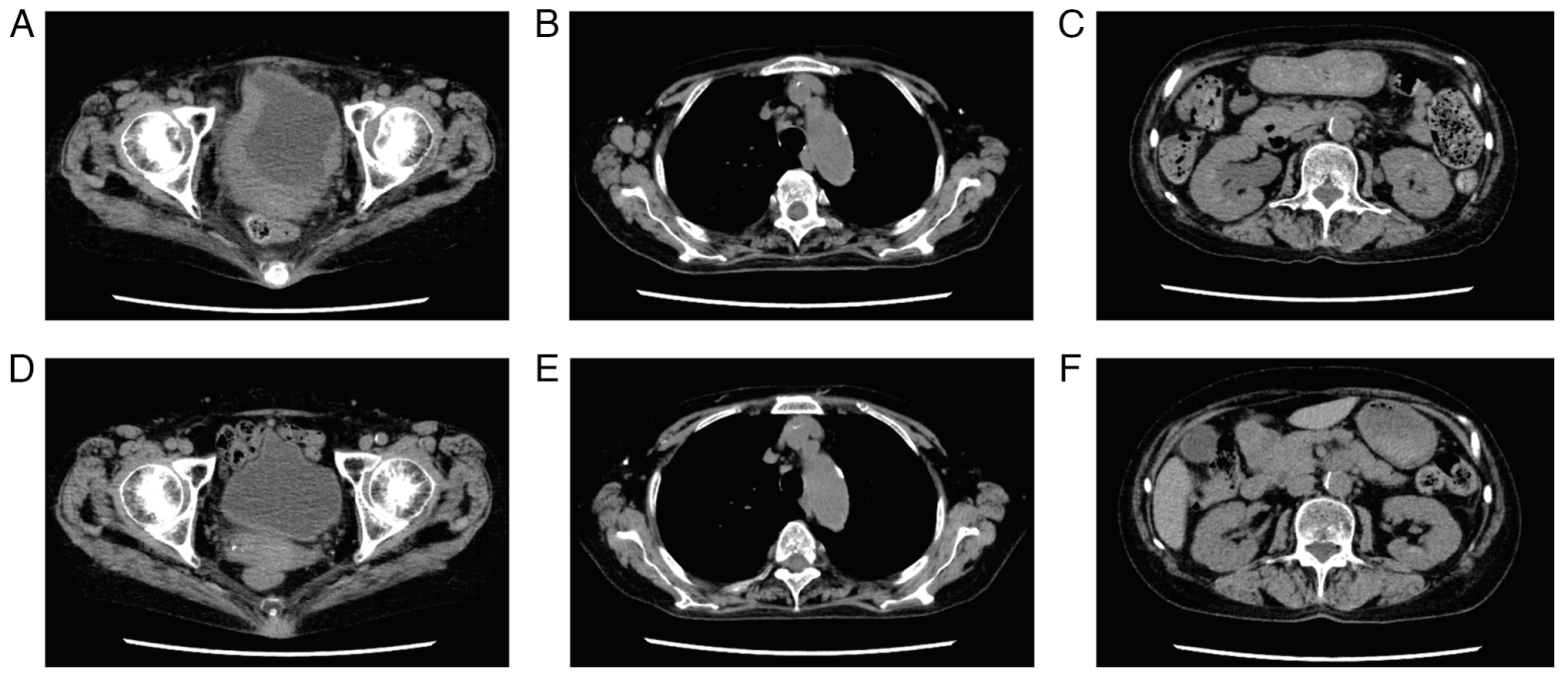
Computed tomography images demonstrating (A) irregular thickening of the urinary bladder wall, (B) swelling of the axillary and mediastinal lymph nodes, and (C) hydronephrosis. Following six cycles of chemotherapy, (D and E) the bladder wall thickening and lymph node swelling had disappeared, and (F) the hydronephrosis had improved.
